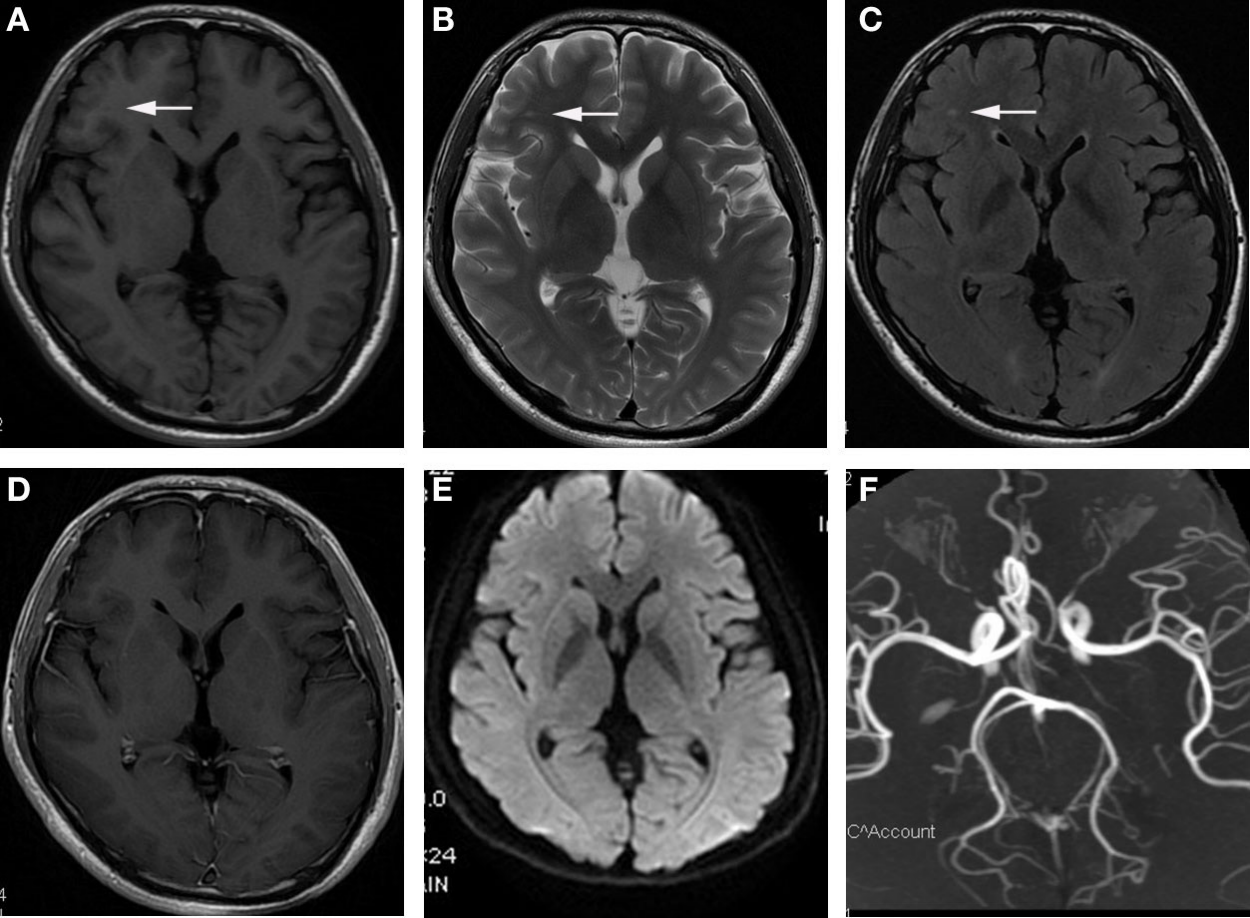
Representational serial magnetic resonance imaging findings of juxtacortical small lesion of a patient who complained about headache for 2 months. (A) low signal intensity on T1-weighted image (T1WI) in subcortical region of left frontal lobe (arrowhead); (B,C) high signal intensity on T2WI and T2 fluid attenuated inversion recovery sequences (arrowhead); (D) no enhancement on T1 + C image; (E) without abnormal signal in diffusion weighted imaging (DWI); (F) the normal image of magnetic resonance angiography (MRA).
